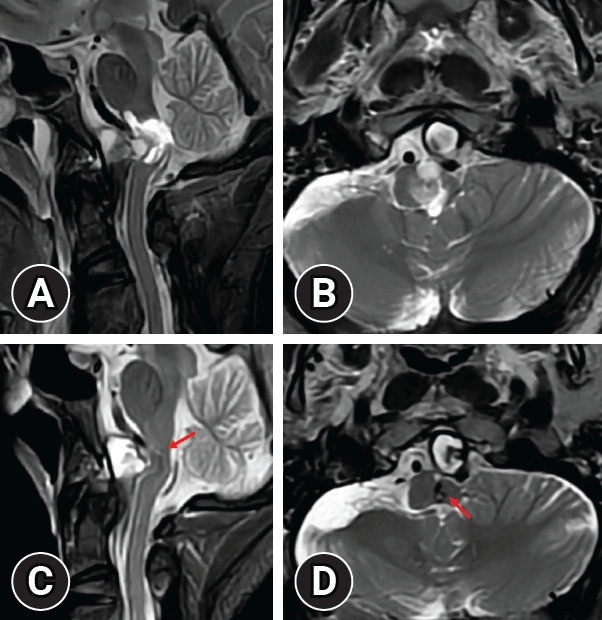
(A, C) Sagittal T2 STIR and (B, D) axial T2-weighted magnetic resonance images. Baseline images obtained at diagnosis (A, B) demonstrate severe bulbar compression by the retro-odontoid cystic lesion. Follow-up images obtained 4 months later (C, D) demonstrate marked reduction in the bulbar edema. Residual focal hypointense signal within the medulla oblongata (arrows) is consistent with hemosiderin deposition related to prior intra-axial hemorrhage. STIR, short tau inversion recovery.
